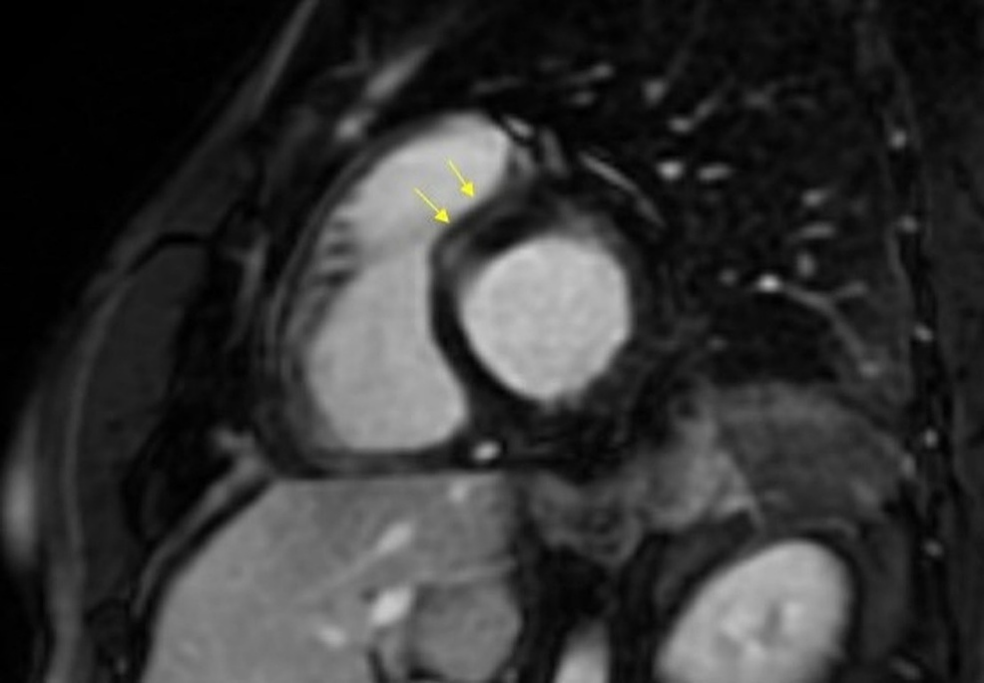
Cardiac MRI with myocardial edema and subtle left ventricular mid-wall late gadolinium enhancement involving the infero septal/inferior wall with preserved left ventricular chamber dimensions and function, consistent with myocarditis.
